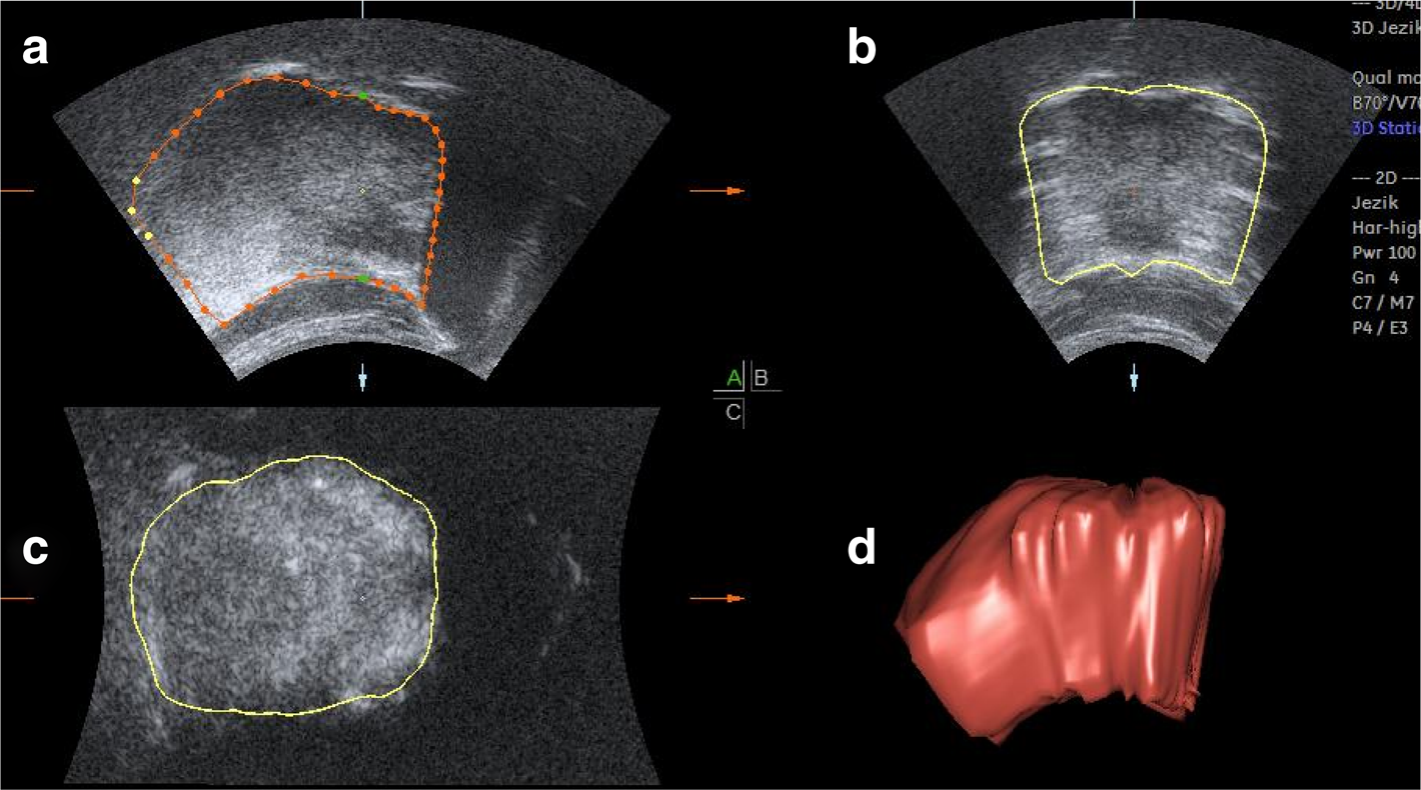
Ultrasound image of the tongue; multi-planar view with boundaries (a sagittal cross-section, b transversal cross-section in dorsum area, c transversal cross-section in apical area, d three-dimensional US reconstruction)
